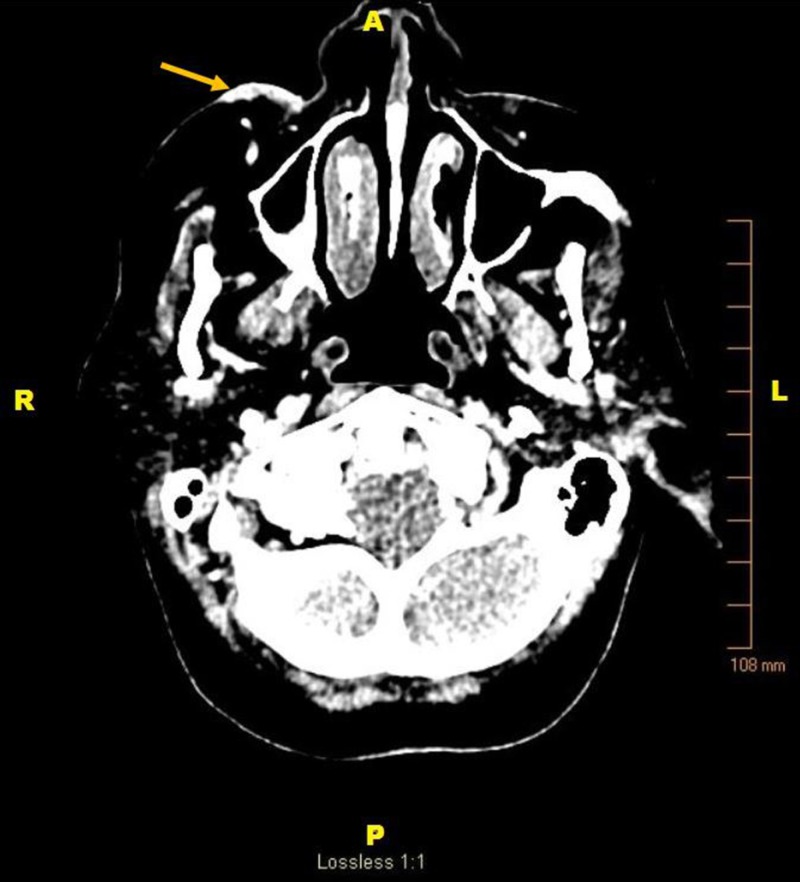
Computed Tomography Head Computed tomography (CT) head with contrast pre-treatment showing (*yellow arrow*) cutaneous thickening and irregularity throughout the right face compatible with angiosarcoma. Legend: A - anterior; P - posterior; R -right; L - left
